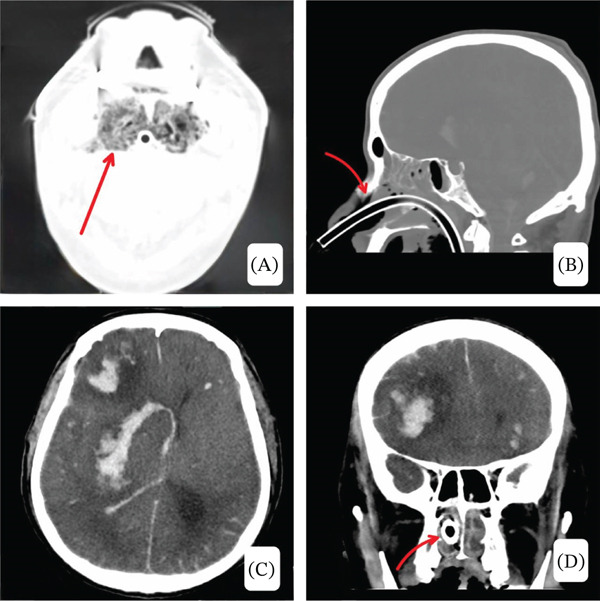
(A–D) (A) Follow‐up CT images demonstrate persistent emphysematous tonsillitis with central and submucosal gas locules, diffuse tonsillar enlargement, and surrounding inflammatory changes. (B) The paranasal sinuses show partial opacification with air‐fluid levels, and the nasogastric tube is clearly visible. (C, D) Multiple intraparenchymal hemorrhages are present supratentorially and infratentorially, with surrounding edema, mass effect, midline shift (~1.3 cm), subfalcine and uncal herniation, and cerebellar tonsillar herniation (~1.1 cm), along with diffuse subarachnoid and mild‐to‐moderate intraventricular hemorrhage and an area of hypoattenuation in the right occipital region. (D) The nasogastric tube is also visible on the coronal image.
